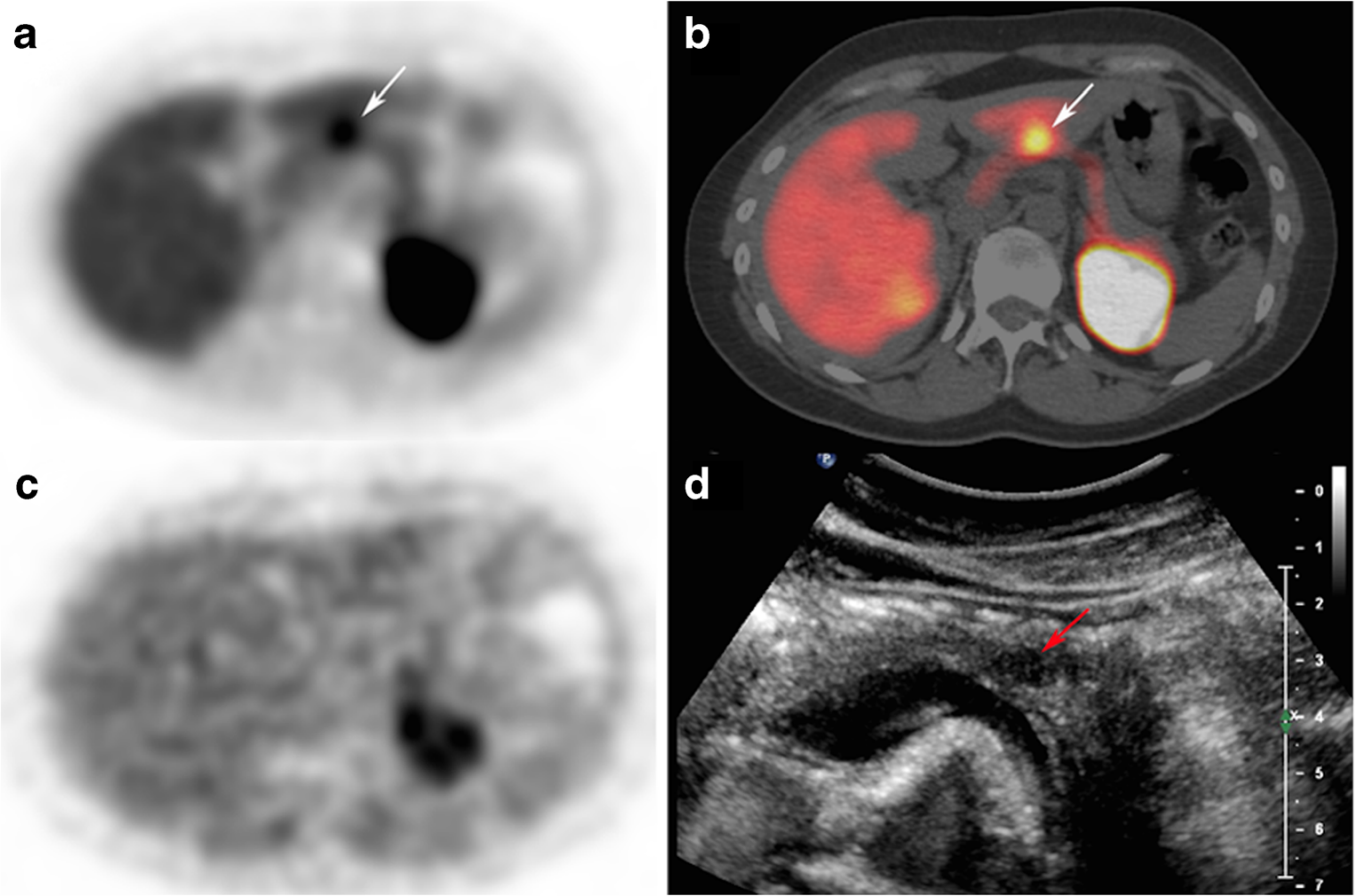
Carbidopa-assisted ^18^F-DOPA PET of a patient suffering from insulinoma. The* arrow* clearly indicates the lesion. a Early PET acquisition. b Axial PET/CT fusion. c Delayed PET acquisition. d Contrast-enhanced ultrasonography [33]
